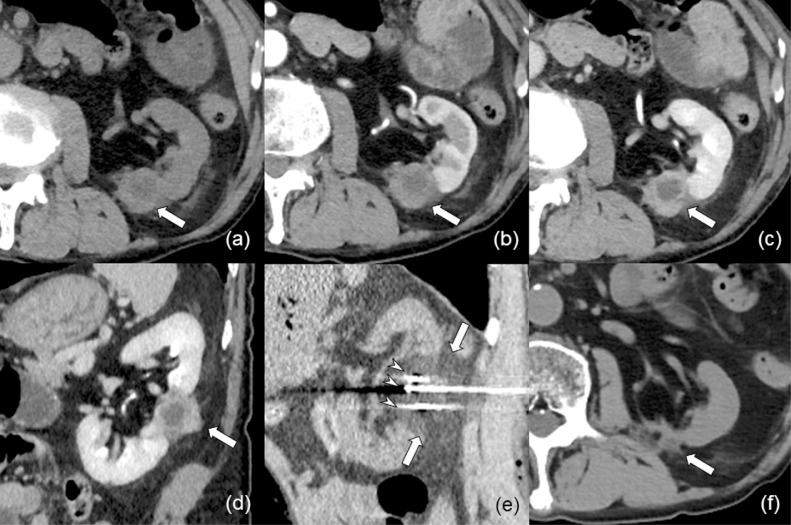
(A-D) Computed tomography (CT) images obtained 4 months after initial cryoablation (CA) confirmed a contrast-enhanced mass (arrow) on the edge of the ablation zone. The mass shows lower enhancement compared with normal renal cortex in both corticomedullary (B) and nephrographic (C) phase, and the mass has a poorly enhanced central part. (D) Sagittal reconstructed CT image on nephrographic phase. (E) Sagittal reconstructed CT image during the second CA shows that the renal mass is surrounded by low attenuation ice ball (arrows) with a sufficient margin. Arrowheads show 3 cryoprobes. (F) CT image obtained 11 months after the second CA shows remarkable shrinkage of the treated area (arrow).
